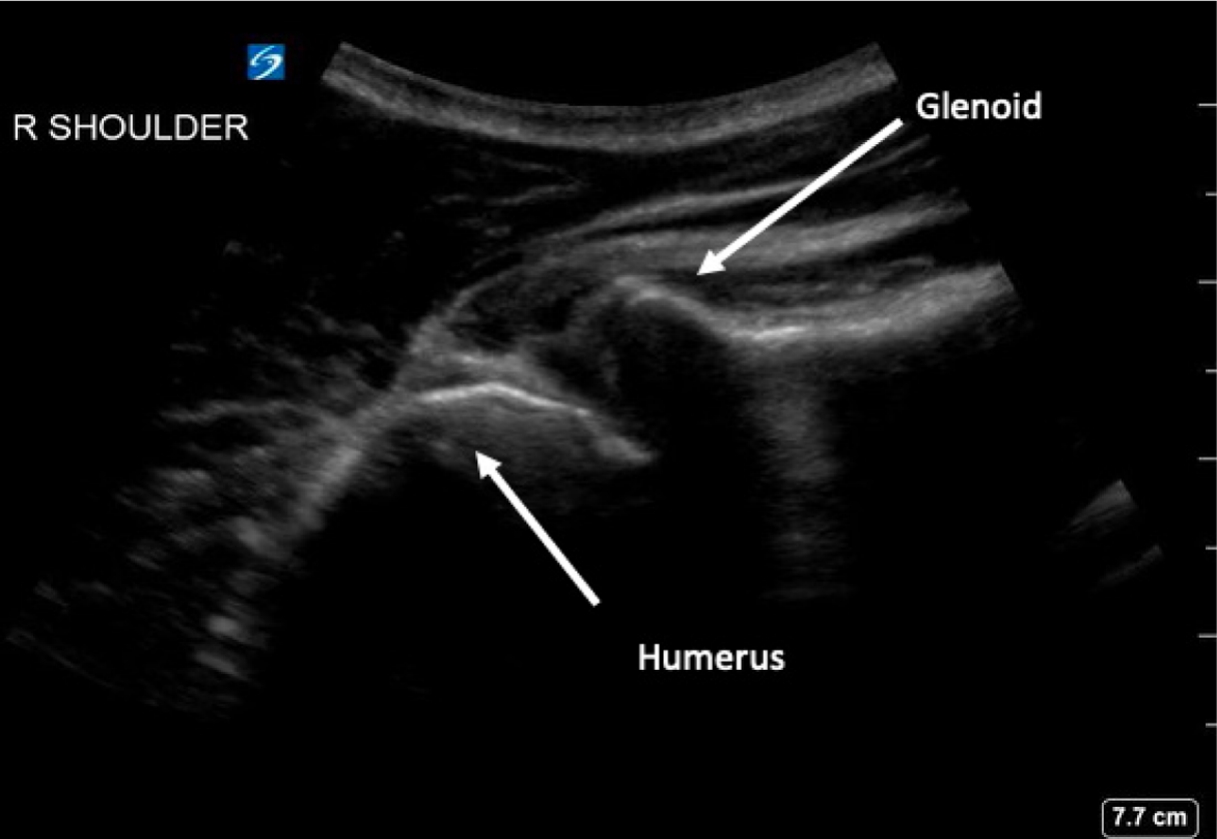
Posterior shoulder ultrasound demonstrating anteriorly dislocated humeral head, courtesy of Michael Rosselli, DO, Mount Sinai Medical Center, Miami Beach.
